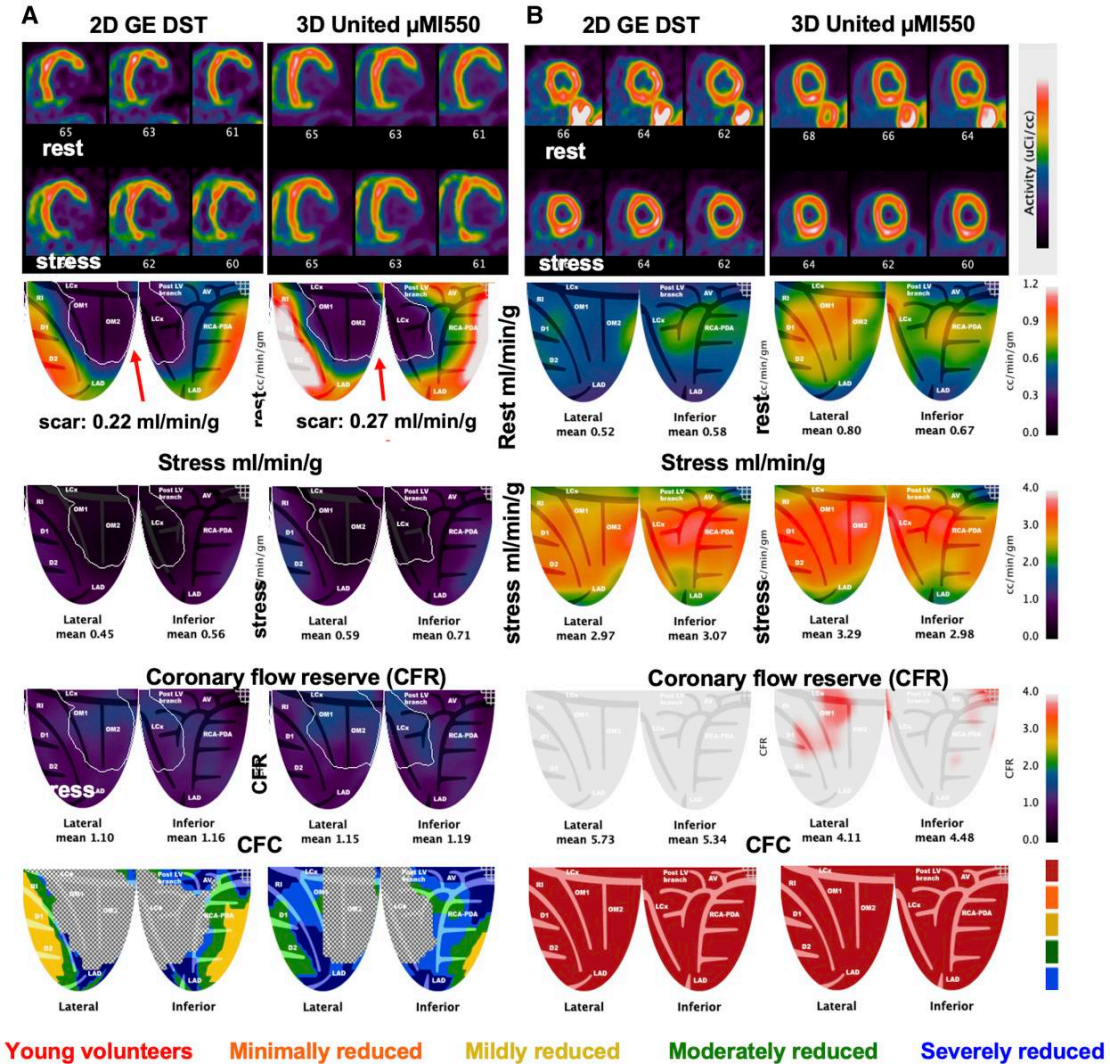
3D United µMI550 compared with established 2D GE DST for extremes of myocardial perfusion. (*A*) A 63-year-old male with known lateral-inferior myocardial infarction (MI), coronary artery bypass surgery (CABG), and PCI with reduced ejection fraction of 35%. Perfusion at rest in the outlined territory of transmural scar (white line) is 0.22 mL/min/g on the 2D DST and 0.27 on the United, respectively. (*B*) A 30-year-old male participant without risk factors or a family history of heart disease shows reproducibility between 2D and 3D PET-CT of rest and stress millilitres per minute per gram and CFR > 4 cc/min/g.
